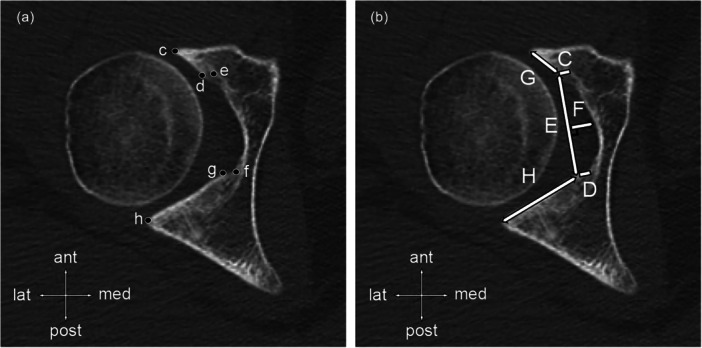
Computed tomography (CT) landmarks and measurements are in reference to the axial hip plane. (a) CT landmarks are shown in c as the anterior border of the anterior lunate surface, d as the posterior border of the anterior lunate surface, e as the anterior edge of the acetabular fossa, f as the posterior edge of the acetabular fossa, g as the anterior border of posterior lunate surface, and has the posterior border of the posterior lunate surface. (b) CT distances measured in this study are shown in C as anterior lunate wall height, D as posterior lunate wall height, E acetabular notch width, F acetabular fossa depth, G as anterior lunate surface, and H as posterior lunate surface.
